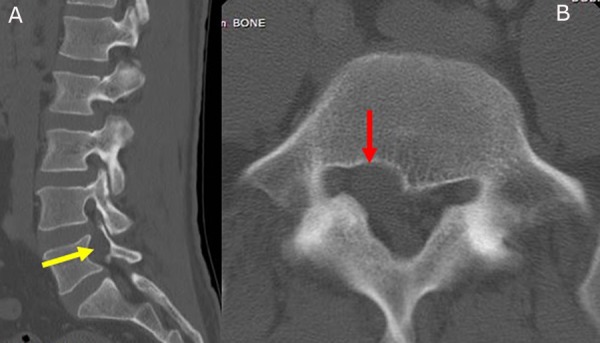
A) The right parasagittal computed tomography (CT) image showing the expansion of the neural foramen (yellow arrow). B) The axial CT scan showing the mass effect of the cyst resulting in the bone resorption and enlargement of the foramen (red arrow).
